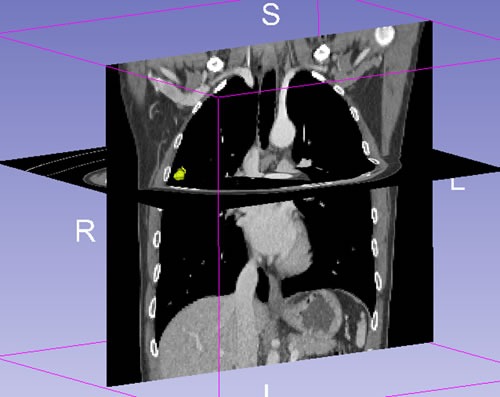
Example of CT images showing segmentation of lung tumor Semiautomatic tumor segmentation was done on every slice of the tumor using 3D slicer (a), and the 3D view of the segmented tumor (b) which was shown in yellow.
